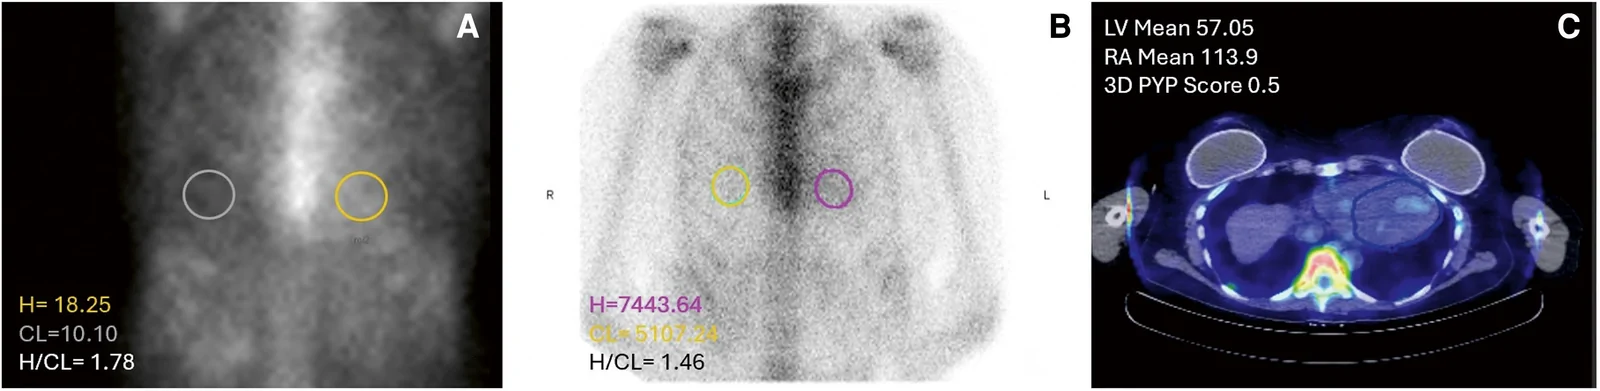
PYP scintigraphy. (*A*) Planar 1-h imaging at an outside facility showed an H/CL ratio of 1.78 (positive ≥ 1.5). (*B*) Repeat planar imaging at 3 h demonstrated an H/CL ratio of 1.46 (positive > 1.3). (*C*) Corresponding SPECT/CT revealed no myocardial uptake, with a 3D PYP score of 0.5 (positive>1.1), confirming the absence of cardiac amyloid uptake. SPECT, single-photon emission computed tomography; PYP, planar technetium-99m pyrophosphate; H/CL, heart-to-contralateral lung.
